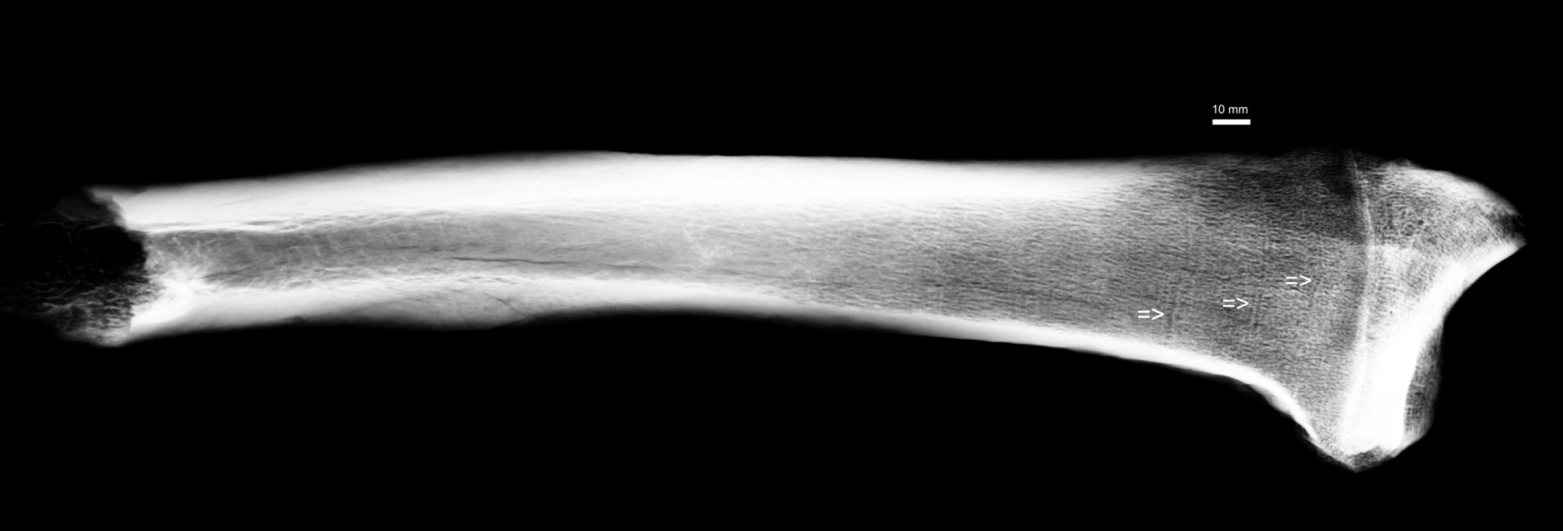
X-ray of radius N° 481/77. Harris Lines indicated with arrows.
